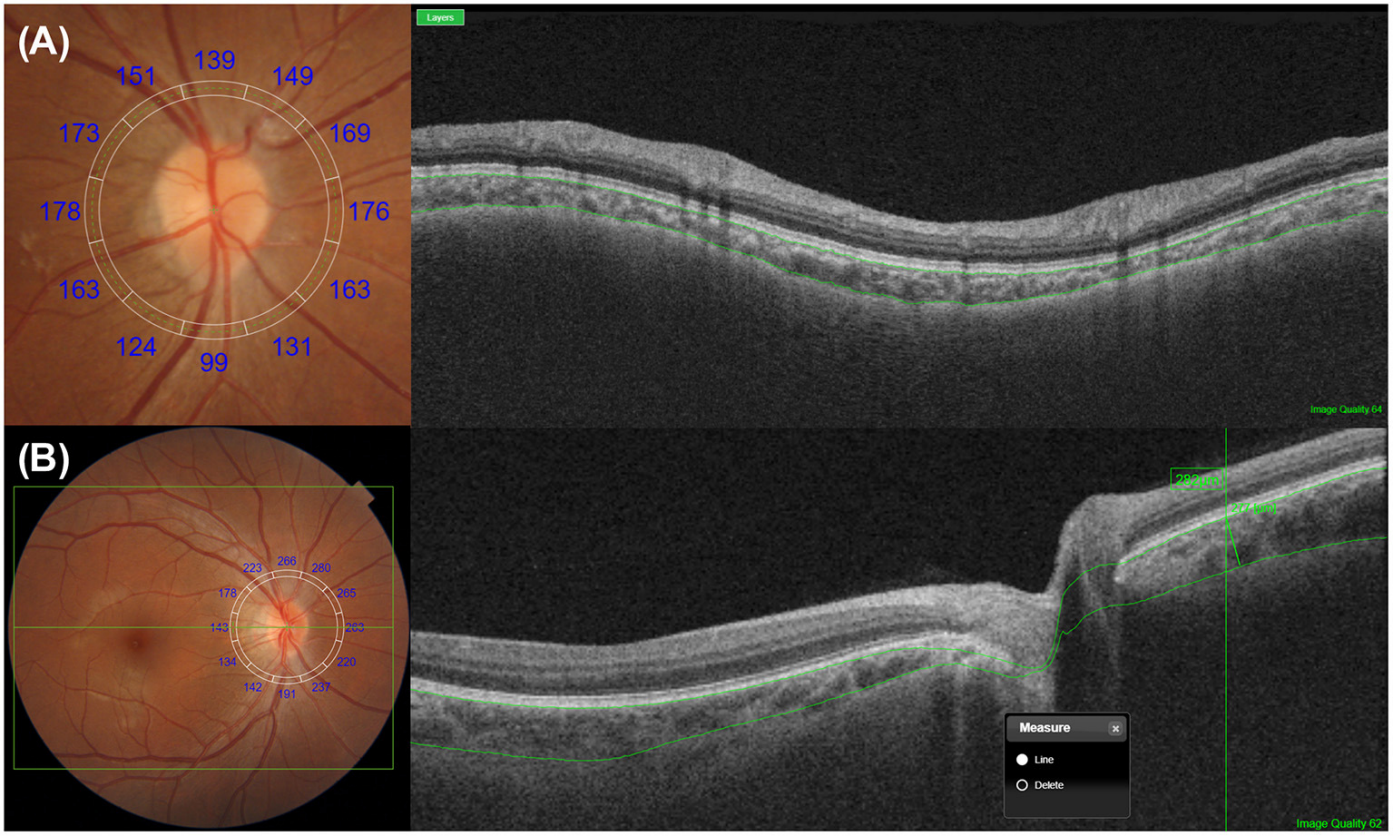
Representative methods for the measurements of peripapillary choroidal thickness (CT). (A) Peripapillary CT can be measured over 360° along a 3.4-mm circular grid, which is used for retinal nerve fiber layer analysis. Depending on the devices, the upper and lower segmentation lines are either automatically or manually selected as the Bruch's membrane and the choroidoscleral junction, respectively. After segmentation, peripapillary CT is determined automatically using built-in manufacturer's software, either by the sectorial or overall average. (B) Peripapillary CT can be measured manually at a point of interest with a similar method for macular CT measurement. A circular RNFL grid centered on the optic disc can be used to improve the consistency of the measurement position.
